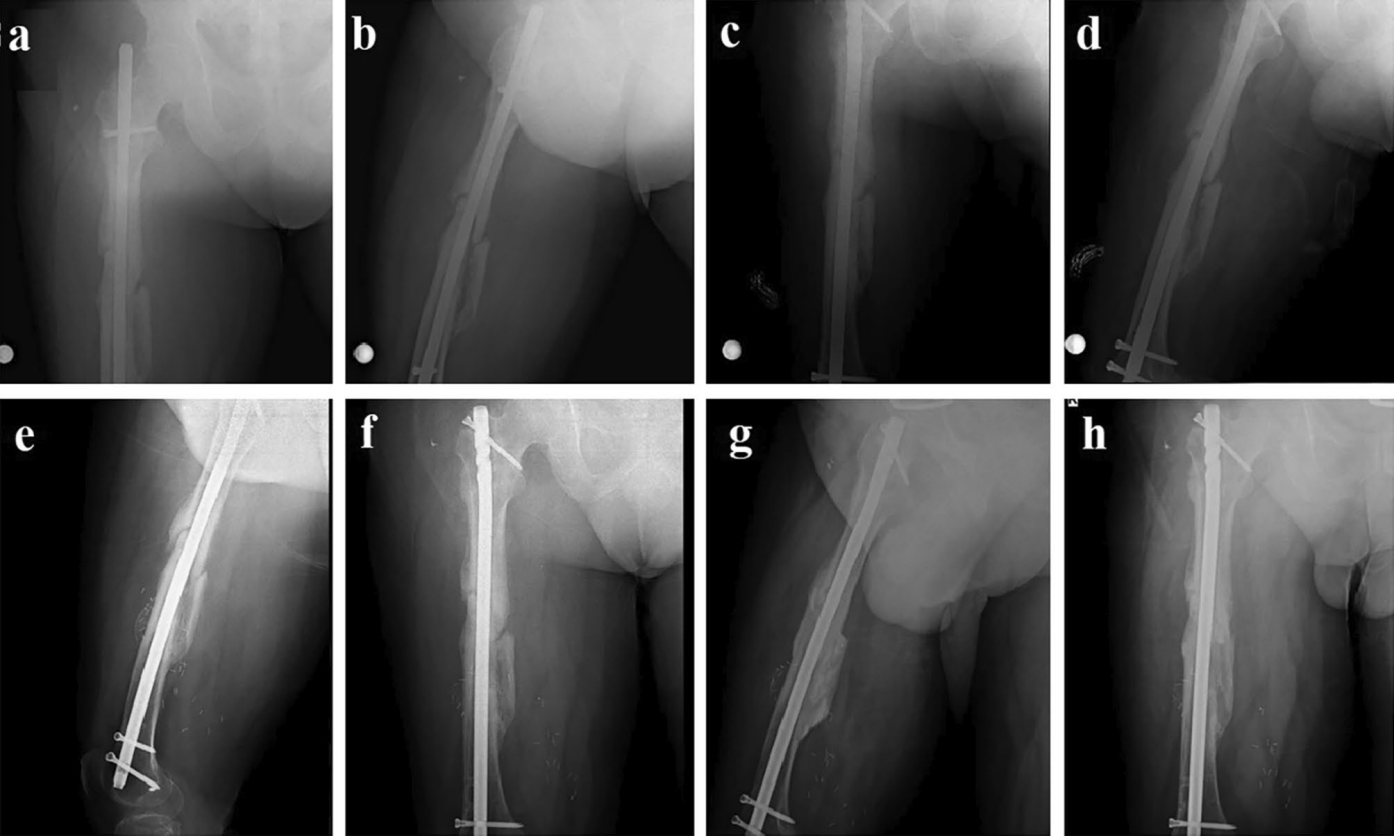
Preoperative nonunion radiographs (a and b), first step treatment: Exchange nailing (c and d), pedicled osteoperiosteal flap surgery (7 months later after exchange nailing) (e and f), postoperative 6th months union radiographs (6 months later after MFC flap) (c and d).
